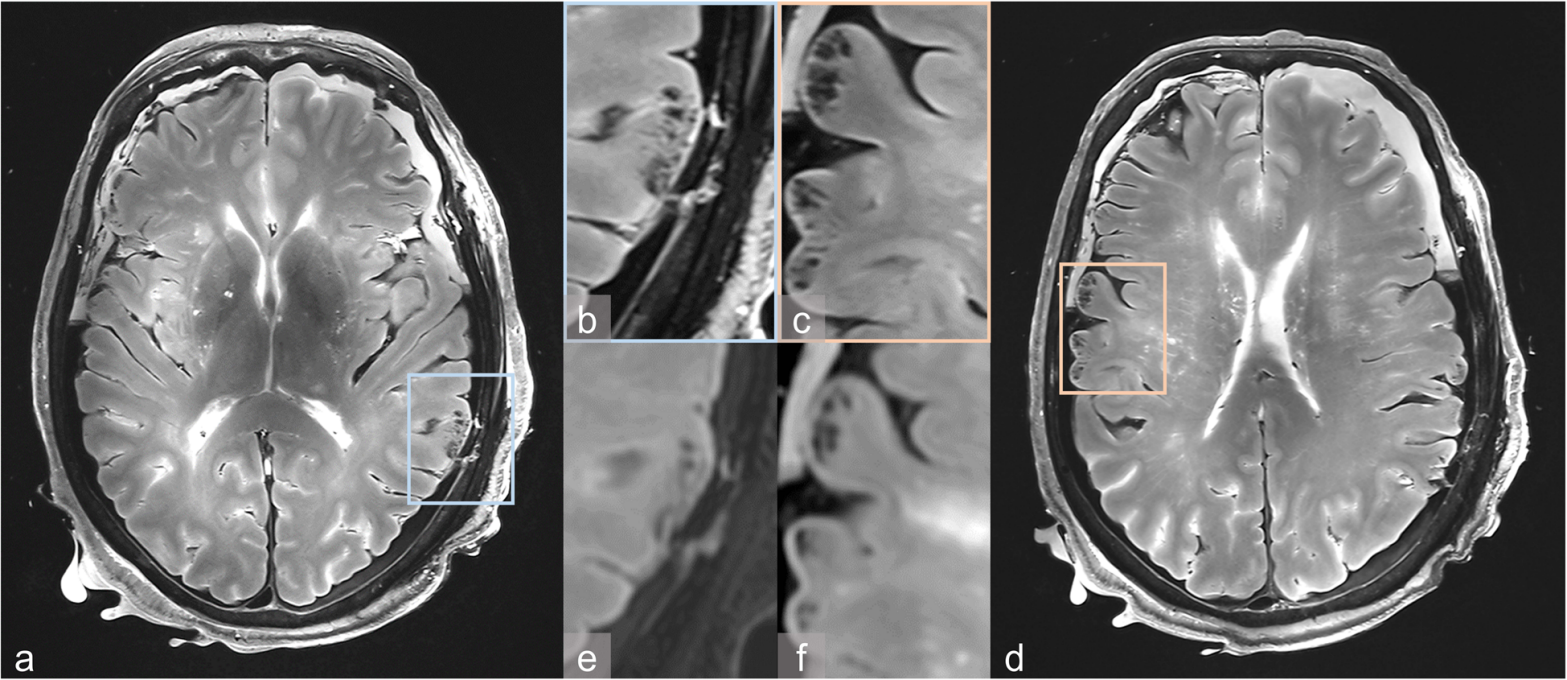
a-d Case 1. 7-T T2-weighted images demonstrate coup injuries (a, b) and contrecoup injuries (c, d). 3-T T2-weighted images appear blurred for both coup injuries (e) and contrecoup injuries (f)
